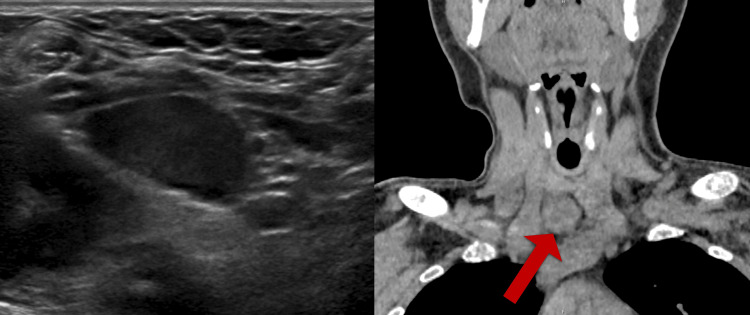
Thyroid ultrasound (left) and CT of the parathyroid scan (right; arrow) revealing right inferior thyroid nodule
